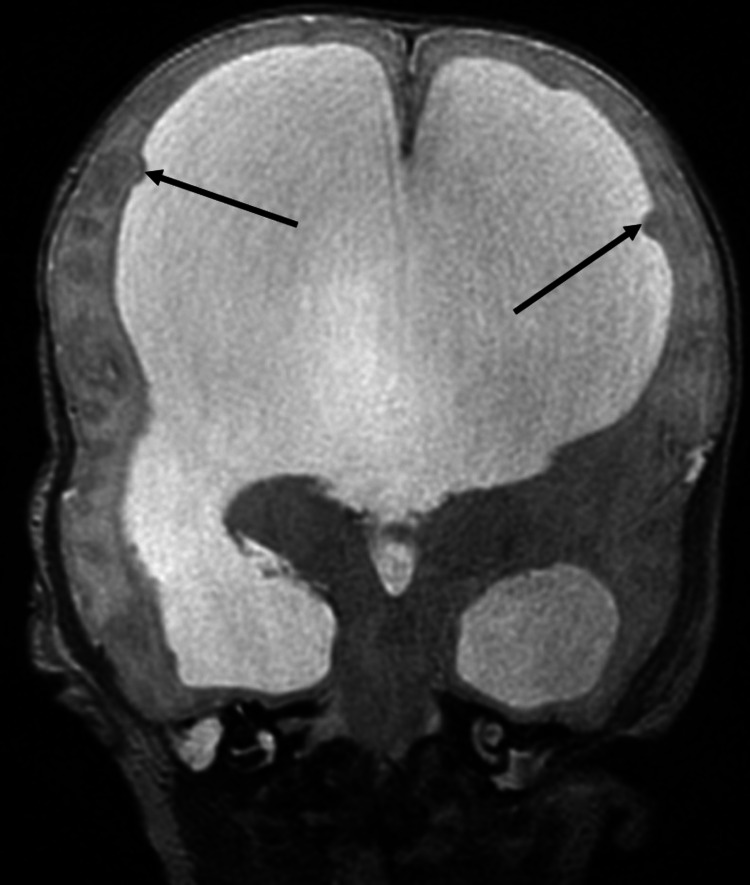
Neonatal MRI: Heterotopia Coronal T2-weighted MRI image demonstrates severe dilatation of the lateral ventricles. Multiple gray matter heterotopias (arrows) that are isointense to gray matter are seen along the lining of lateral ventricles.
